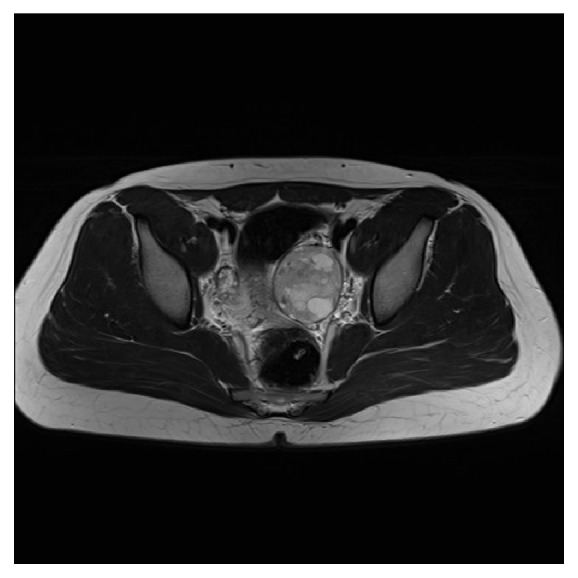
MRI image of ovarian lesion.
